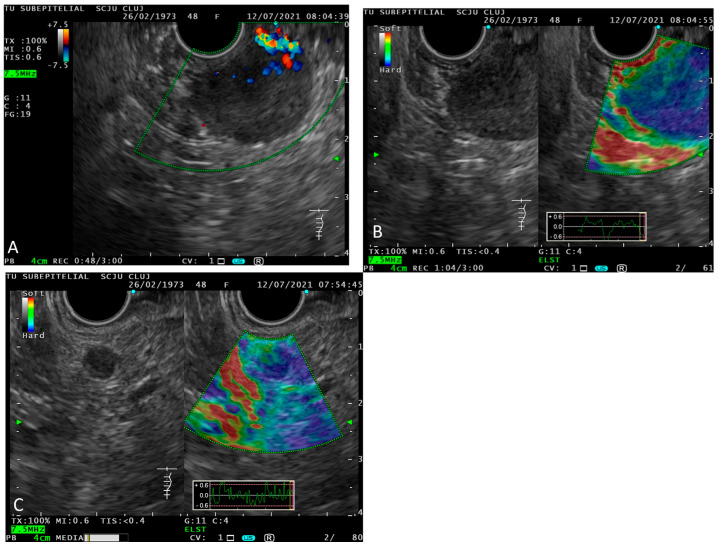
EUS periampular tumor in US, Doppler-US and elastography showing the vascular pedicle and increased rigidity of the tumoral mass (A,B); EUS periduodenal lymph node: image in (B) mode and elastography showing increased nodule rigidity (C).
